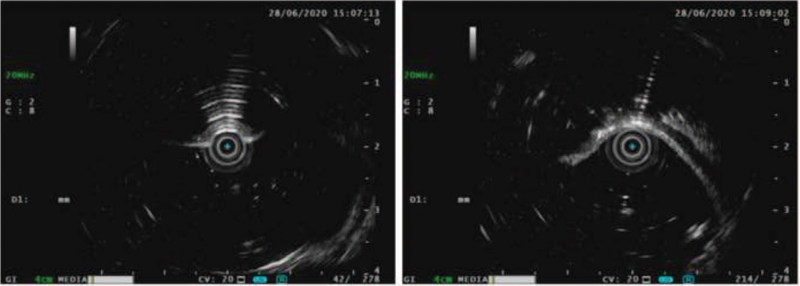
Endoscopic ultrasound with 20 MHz-probe showing hyperechoic air pockets in the submucosa of the ascending colon with ringing artifacts.
